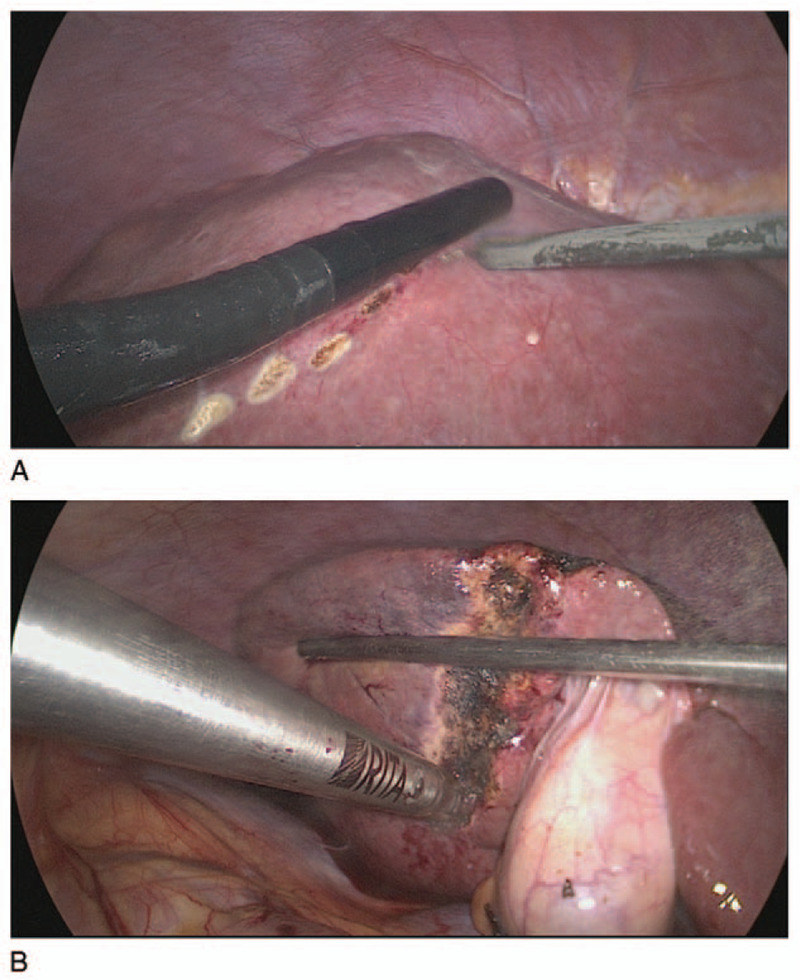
(A) A line for liver partition is made on the surface of the liver under ultrasound guidance. (B) Avascular necrosis along the future line of transection is produced by using the Habib Sealer (LH4X, Rita).
